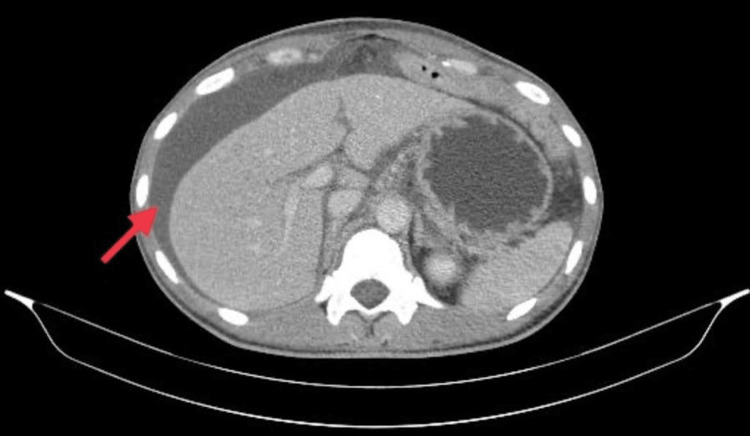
CT scan of the abdomen demonstrating peritoneal fluid
